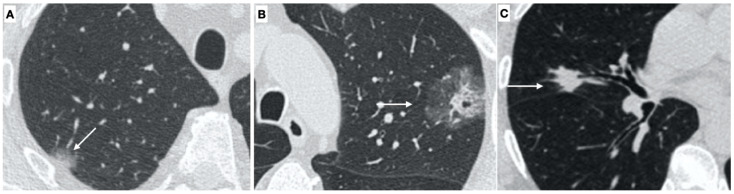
(A) High resolution CT scan shows a ground glass opacity in the right upper lobe (arrow); a minimally invasive adenocarcinoma with predominant lepidic growth was diagnosed. (B) High resolution CT scan demonstrates a partial solid nodule in the upper left lobe, with a solid component of 9 mm (arrow) and a final diagnosis of adenocarcinoma. (C) A solid nodule of 12 mm in the right upper lobe with (arrow) a final diagnosis of squamous cell carcinoma.
